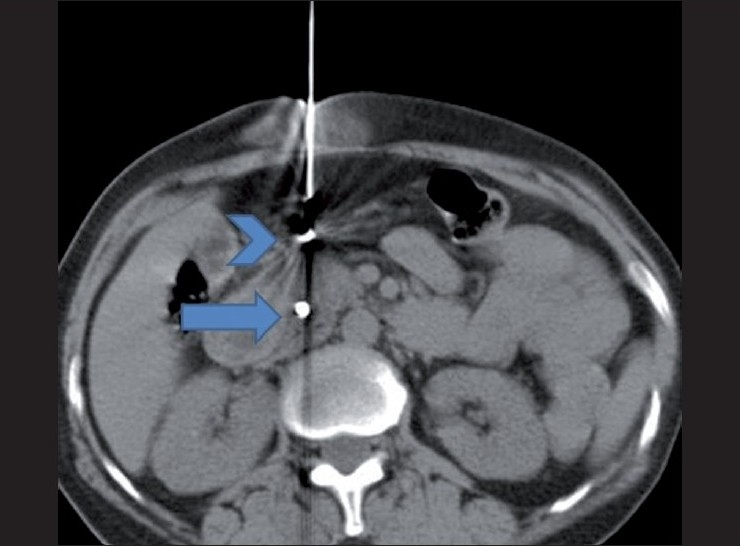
Axial CT scan after placement of a fiducial (arrow) in the pancreatic head and withdrawal of the needle (arrowhead) to change its direction
